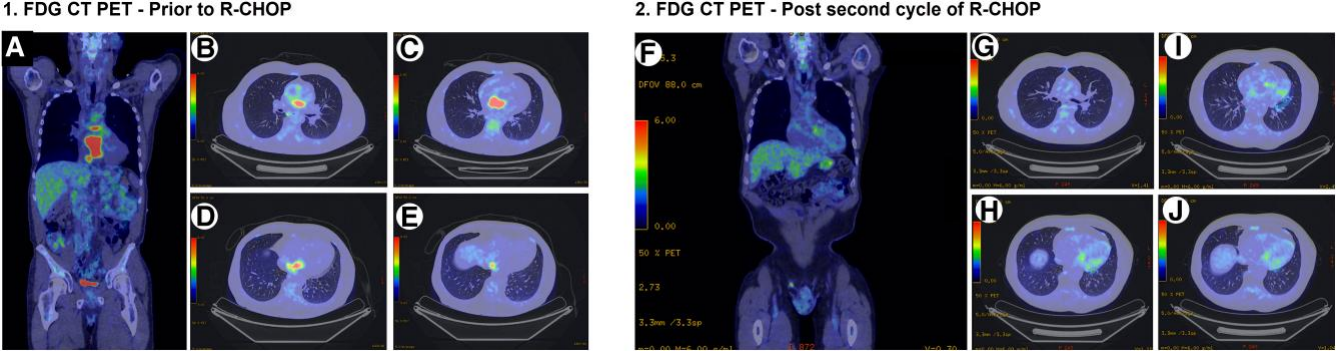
(1) CT PET scan showing marked uptake in the heart. (1*A*) Coronal whole body FDG PET showing areas of avidity highlighted in red. 1*B–E*. Areas of avidity are shown in red extending from the IVC through the intra-atrial septum cranially. (2) CT PET showing reduced avidity. (2*F*) Coronal slice showing reduced avidity compared to the pretreatment scan. (2*G–J*) Axial slices from the precarinal level to the IVC showing marked reduction in avidity throughout the heart and surrounding structures.
